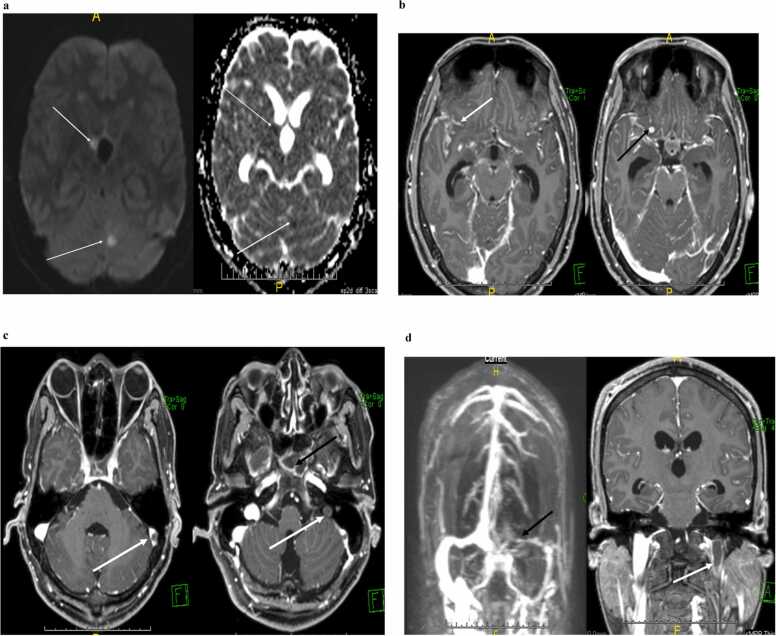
a: MRI of the brain showing a paired Diffusion weighted (DWI) sequence with an apparent coefficient map (ADC map) sequence indicating the vasculitic infarcts (white arrows). Note that they appear hyperintense on DWI and hypointense on ADC map indicating acuteness. b: Axial contrast enhanced T1 weighted MRI of the brain showing ring enhancing right frontal tuberculoma (black arrow). Note the accumulation of inflammatory exudates around the right Sullivan fissure in a gyral pattern (white arrow). c: Axial T1 contrast-enhanced MRI of the brain showing thrombosis of the right sigmoid sinus extending down to the jugular vein (white arrow). Note the intense basal enhancement (black arrow). d: Contrast enhanced MRV (left) showing filling defect in the left transverse and sigmoid sinuses as extending well into the left jugular vein (black arrow). Coronal contrast enhanced MRI of the brain: showing the extension of the thrombus well into the left jugular vein (black arrow).
